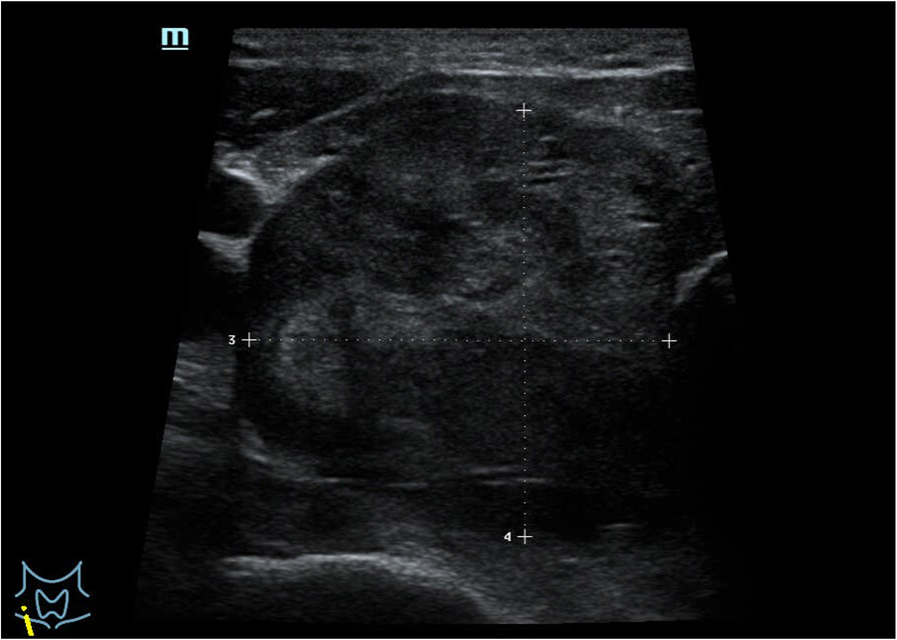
Ultrasonography showed a right thyroid mass
